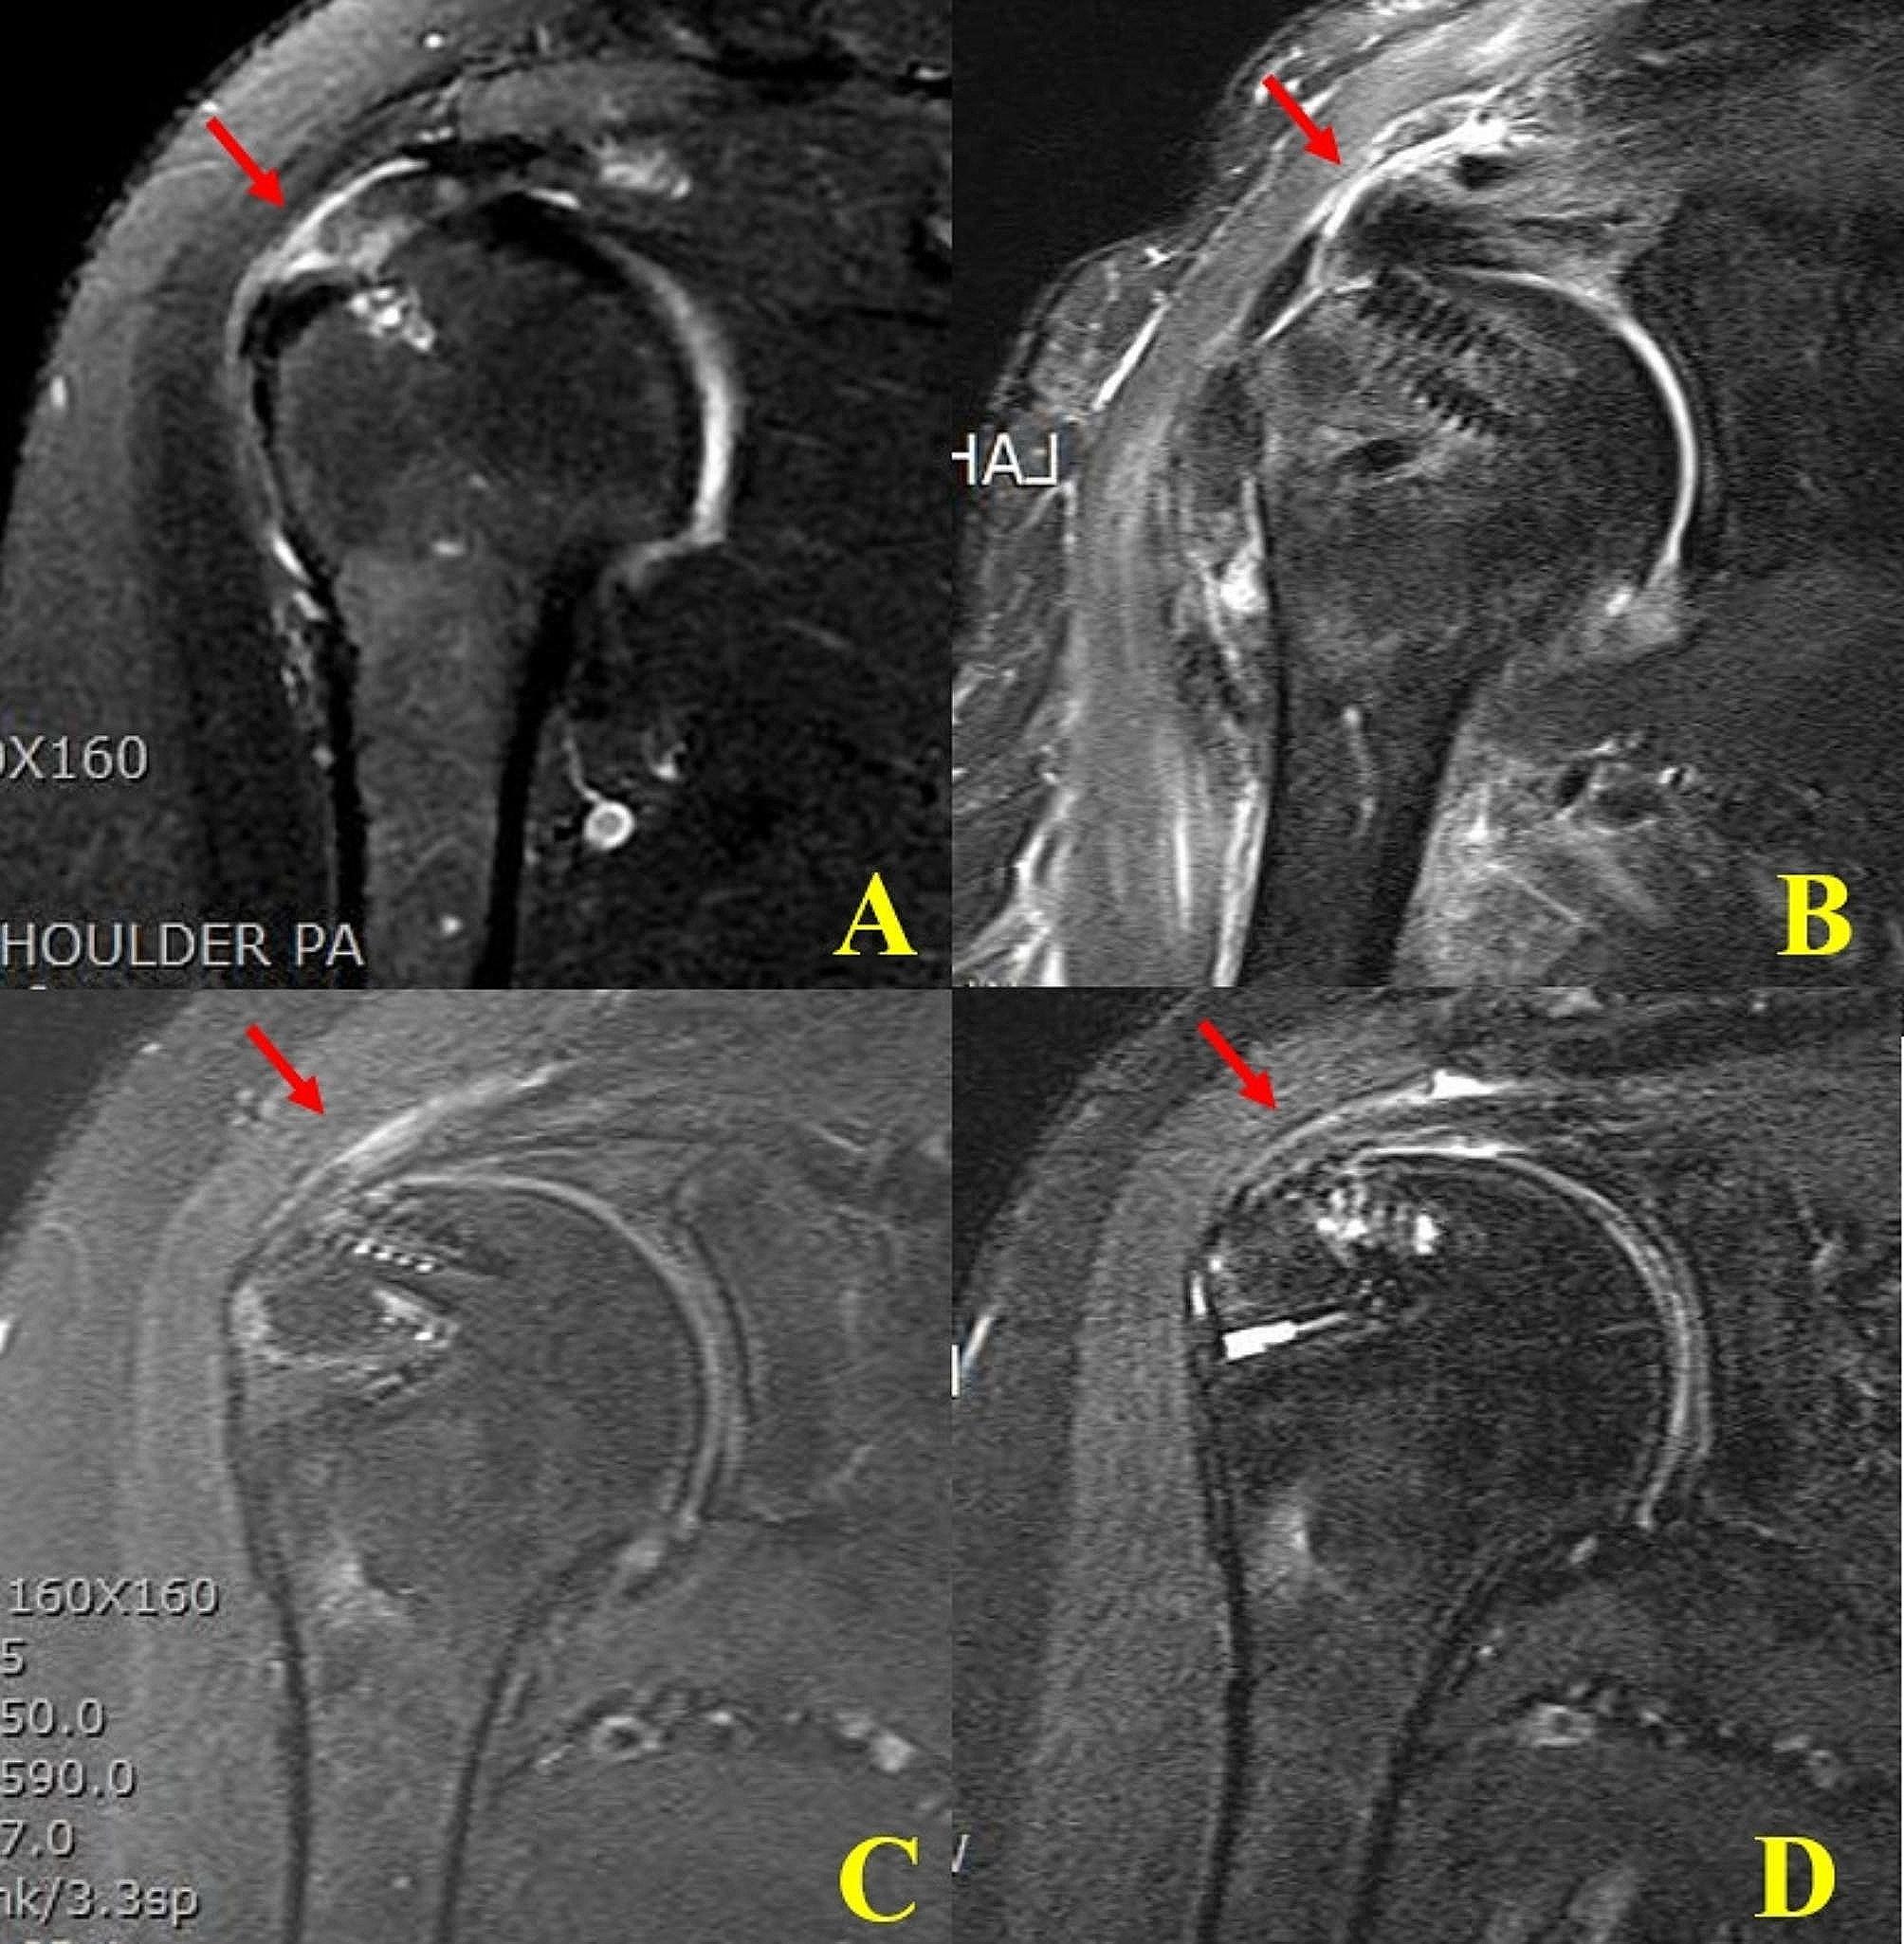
The preoperative and postoperative MRI scans. (A) The oblique coronal FSE T2-weighted image clearly showed the presence of the footprint remnant tendon in the transtendinous full thickness rotator cuff tears (arrow). (B), (C), (D) Immediate, postoperative 1, and postoperative 6 months follow-up MRI showed intact cuff integrity without high signal intensity (arrow)
